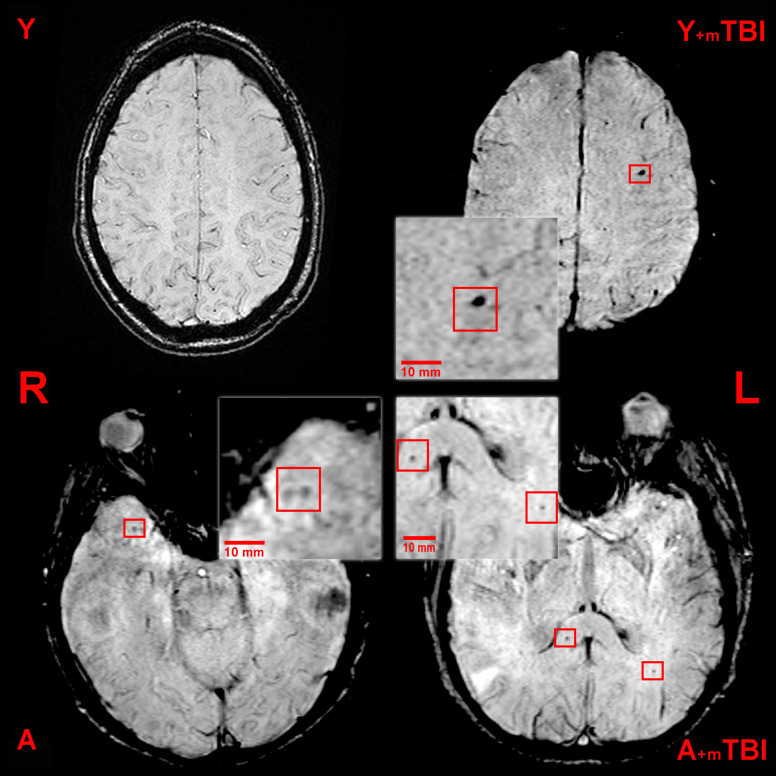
Axial susceptibility weighted (SWI) MRI (three Tesla) of a young control patient (Y, 38-year-old, male), a young patient following mild traumatic brain injury (Y + mTBI, 36-year-old male, GCS: 15), an aged control patient (A, 67-year-old male), and an aged patient with a mild TBI (A + mTBI, 65-year-old male, GCS: 15). Cerebral microbleeds (CMBs) appear as ovoid, hypointense lesions and are indicated by the red squares (R, right; L, left).
